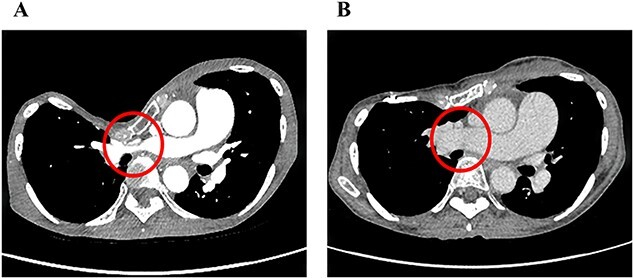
Pre- (A) and postoperative (B) chest CT findings of severe PE with stenosis of the right main pulmonary artery. The diameter of the right main pulmonary artery increased from 5 to 20 mm after the CRN procedure.
